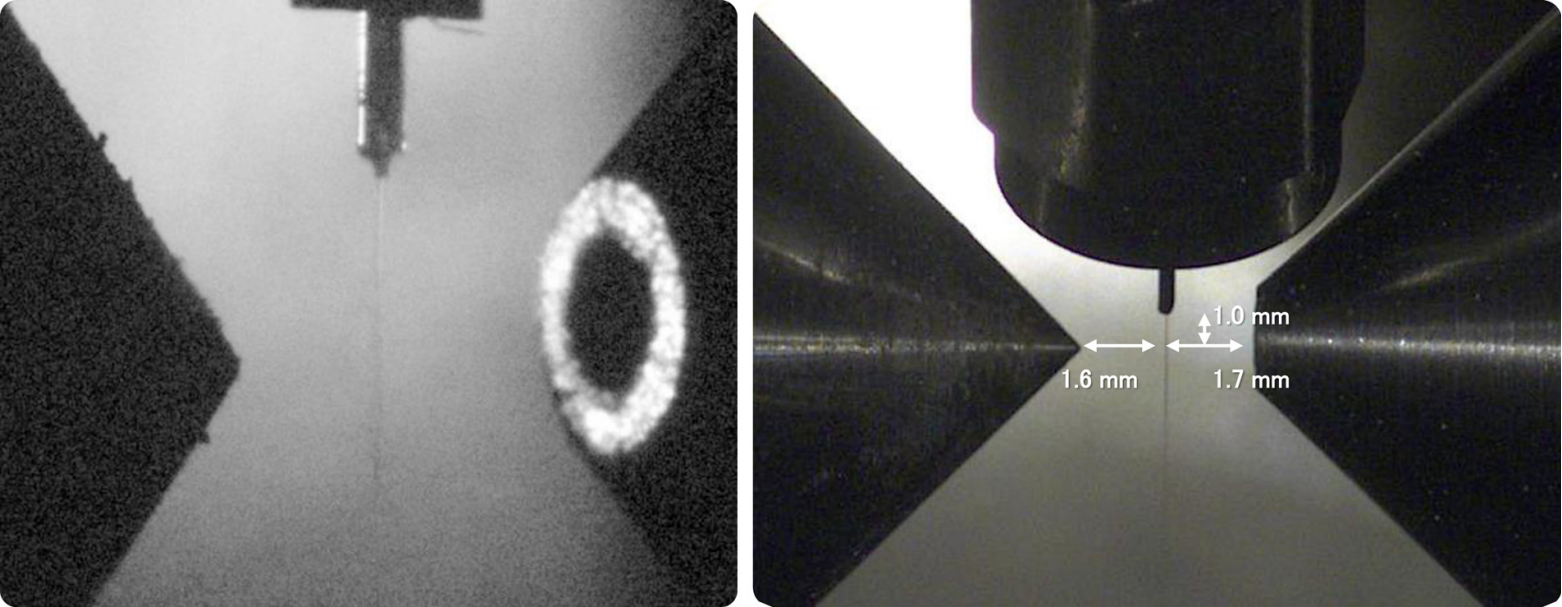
The liquid jet between the permanent magnet (to the left in both images) and skimmer orifice (to the right) is observed from different directions via CCD cameras; here, the side view aligned 135° to the TOF axis (left image) in the horizontal plane and the top view elevated 45° above the X-ray propagation axis (right image) is shown.
